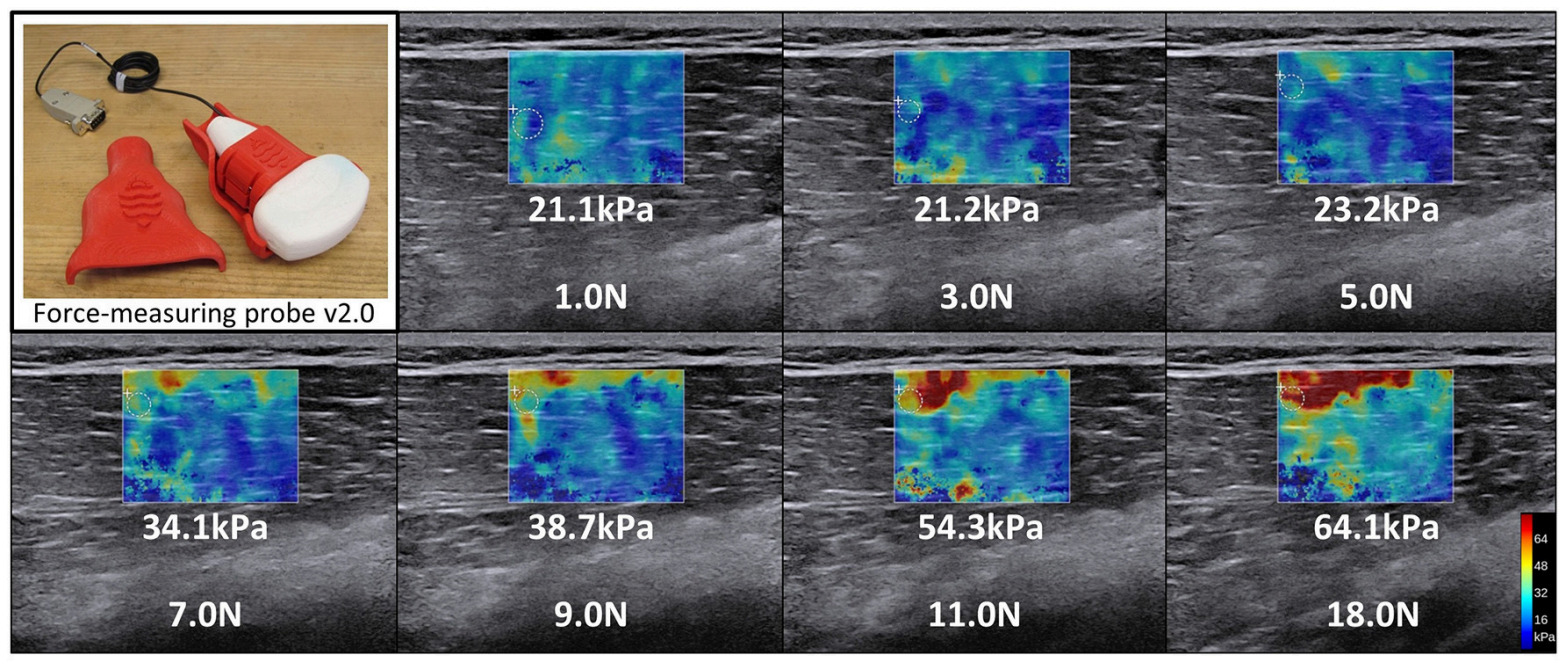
Variation in shear wave elastography secondary to the applied scanning force. Significant variation in shear wave elastography estimates of tissue Young's modulus shown in the figure is a function of preload differences typical of clinical sonography. The varying preload conditions depicted are typical of those seen across a range of operators in routine abdominal sonography and the resultant change in estimated tissue Young's modulus. This variation is explained by the observation that different bias compression levels pre-strain the tissue to different operating points along the tissue's non-linear stress-strain response. Estimated Young's modulus increases from 21.1 to 64.1 kPa in the vastus medialis as applied force (preload) increases from 1 to 18 N.
